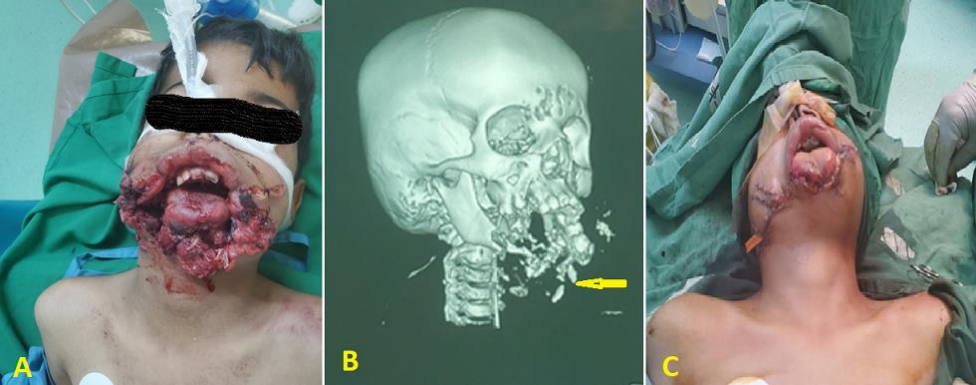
A) facial view at the time of initial evaluation; B) 3D reconstruction computed tomography scan showing subtotal destruction of mandibular bone (arrow); C) postoperative photo after approximation of the lower face defect edges
